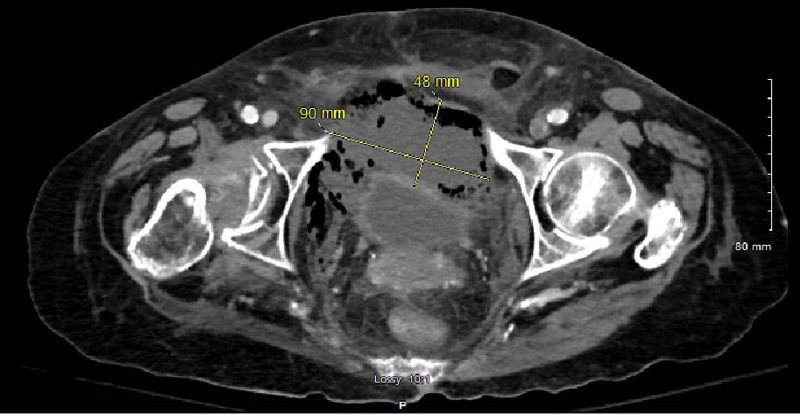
CT scan – axial view – the periappendiceal abscess was approximately 9.0 x 4.8 cm in size
